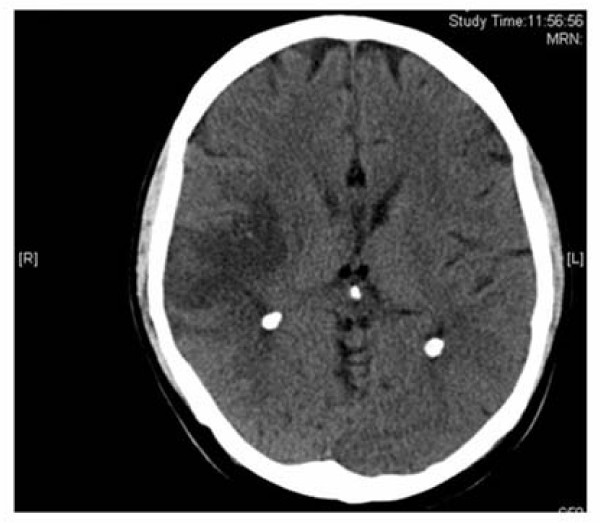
CT demonstrating large ischaemic infarct in the right parietal lobe.
